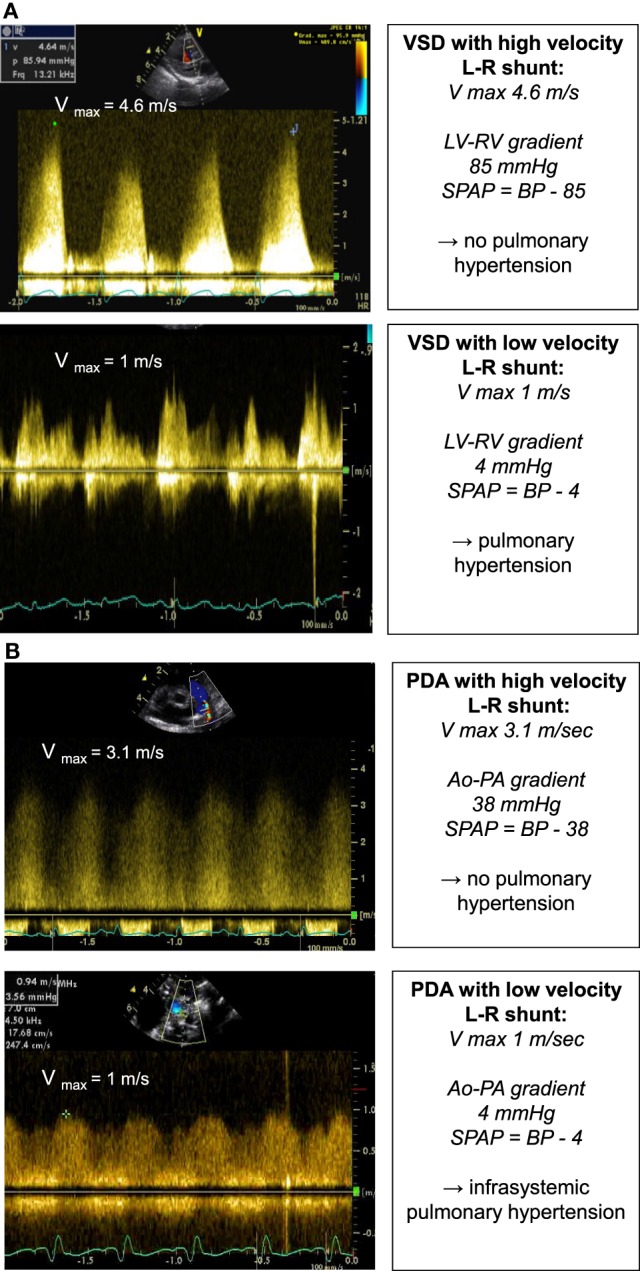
Spectral Doppler (A) obtained through a VSD with high velocity left-to-right shunt and no pulmonary hypertension (above picture) and with low velocity left-to-right shunt and pulmonary hypertension (below picture). The pressure gradient across the VSD allows for estimation of the systolic PAP. Spectral Doppler (B) obtained through a PDA with high velocity left-to-right shunt and no pulmonary hypertension (above picture) and with low velocity left-to-right shunt and pulmonary hypertension (below picture). The pressure gradient across the PDA allows for estimation of the systolic PAP. BP, systolic blood pressure; LV, left ventricle; PAP, pulmonary artery pressure; PDA, patent ductus arteriosus; RV, right ventricle; VSD, ventricular septal defect.
